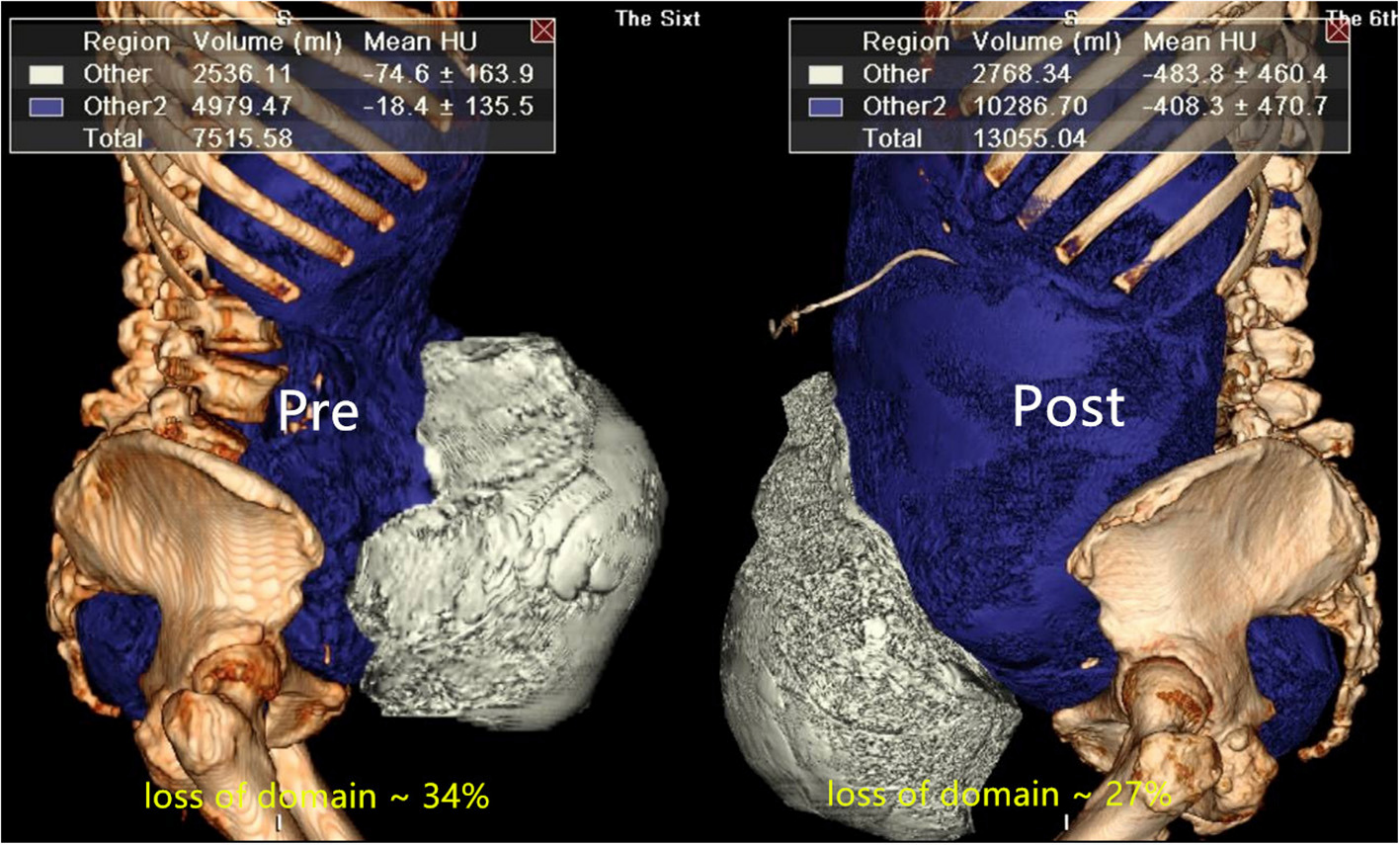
The abdominal volumes (VAC, VIH) were calculated from 64-slice multi-detector CT scan using specific software before and after preoperative techniques.
